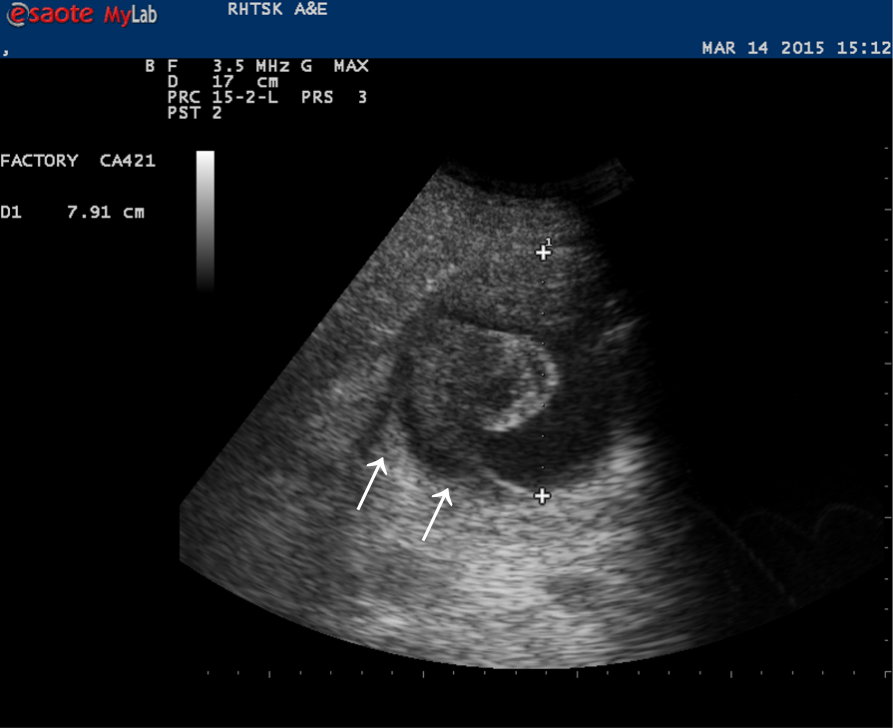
Sonography image showing a 7.9-cm mass with free fluid [←] around it
